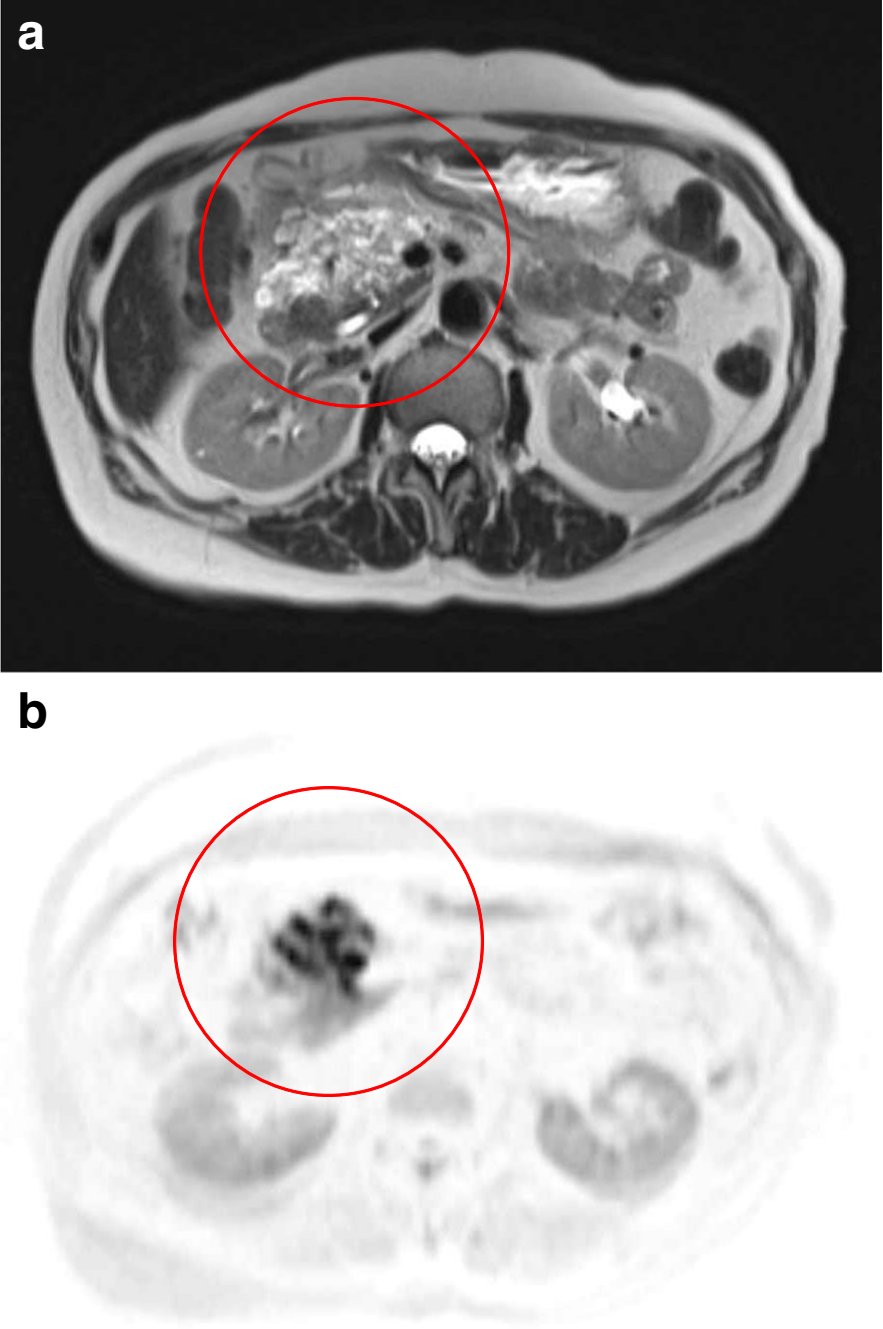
MRI showed a lobulated tumor with mixed high and low signals on T2-weighted imaging (a). Diffusion-weighted images revealed a high signal lobulated tumor (b). (red circle in each image)
